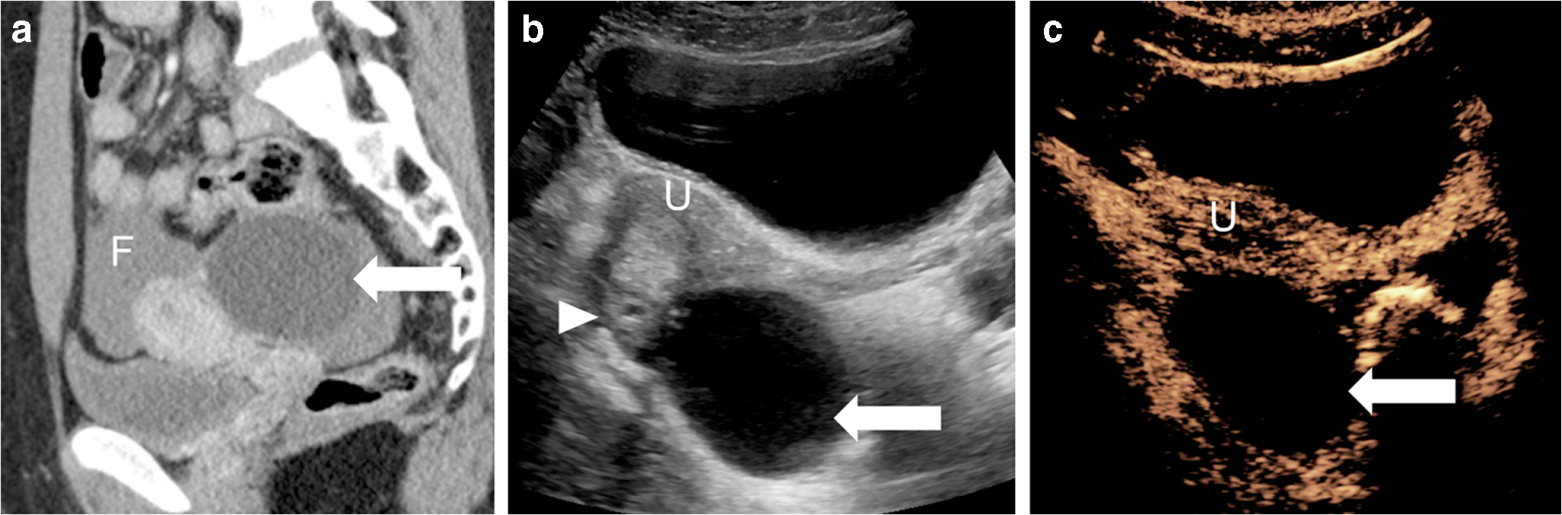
Ovarian cyst in a 16-year-old girl. CT was performed following blunt abdominal trauma. a Sagittal reconstruction from contrast-enhanced CT of the abdomen and pelvis demonstrates hemorrhagic fluid (*F*) in the pelvis from a splenic laceration (not shown here). A large cystic lesion (*arrow*) is noted posterior to the uterus. b Sagittal trans-abdominal gray-scale US demonstrates the anechoic lesion (*arrow*) posterior to the uterus (*U*) in direct contact with the right ovary (*arrowhead*). c Sagittal contrast-enhanced ultrasound (CEUS) of the pelvis in contrast mode shows no visible enhancement, nodularity or septations within the ovarian lesion (*arrow*), compatible with simple cyst
